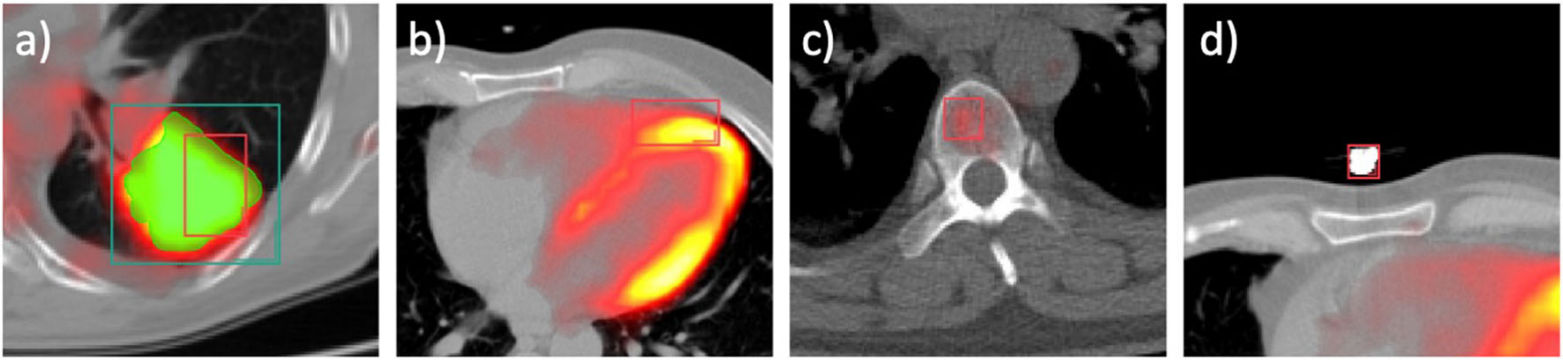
Four examples of FP findings (marked on PET/CT with red bounding boxes) due to (a) a double annotation of a true lung tumor that had already been detected (green bounding box), (b) the physiological activity of the myocardium, (c) the physiological activity of bone marrow, and (d) an extracorporal foreign body
